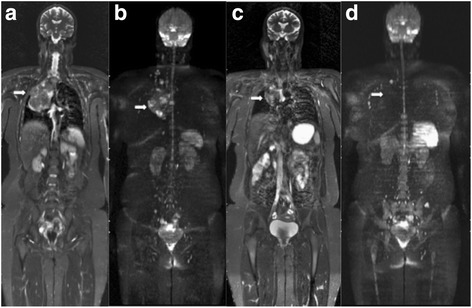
A 16-year-old female patient with primary mediastinal Hodgkin lymphoma (stage III-B) underwent whole-body MRI with STIR (a, c) and diffusion (b, d) sequences. a and b Examinations performed for pre-therapeutic staging showed a voluminous anterior mediastinal lesion (*arrows*). c and d Post-therapeutic imaging showed the presence of a residual mediastinal lesion with no sign of activity (*arrows*)
